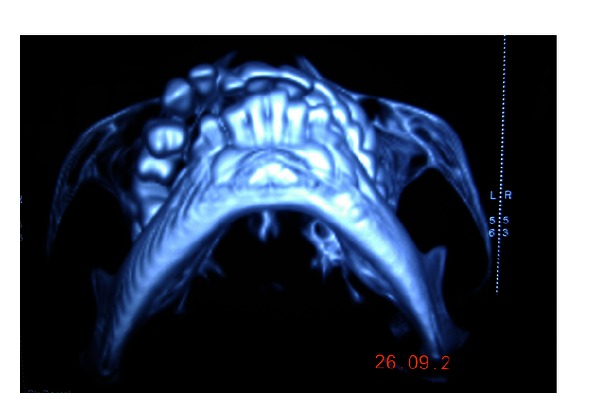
Submandibular view in CT scan showing the right side osseous maxillary alveolar expansion.
